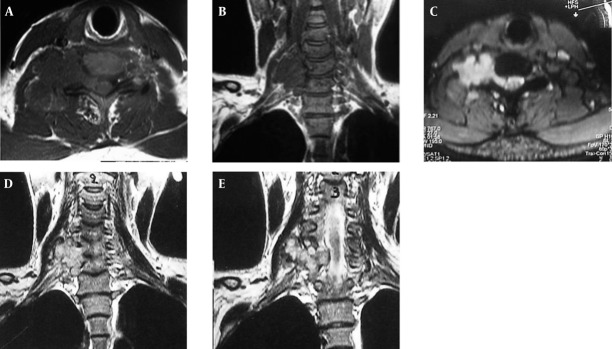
Magnetic resonance imaging of the cervical spinal column with 1.5 Tesla magnet on T1-weighted and T2-weighted sequences with contrast material reveals a lobulated dumbbell-shaped soft tissue mass with regular border originated from the neural arcus of the fifth cervical vertebra extending to the right-sided C4-C5 neural foramina. It has low to intermediate signal intensity on T1-weighted axial (A) and coronal (B) images and high signal intensity on T2-weighted axial image (C) and T2-weighted coronal image (D & E).
